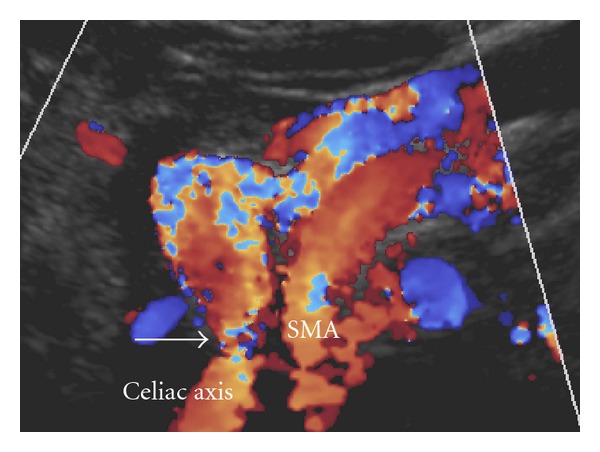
Color ultrasound study at 3-month follow up shows focal residual stenosis at proximal celiac artery.
